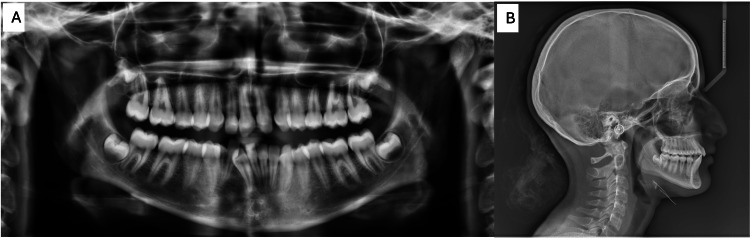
Pre-treatment radiographs. The images show (A) pre-treatment panoramic and (B) pre-treatment lateral cephalogram.
